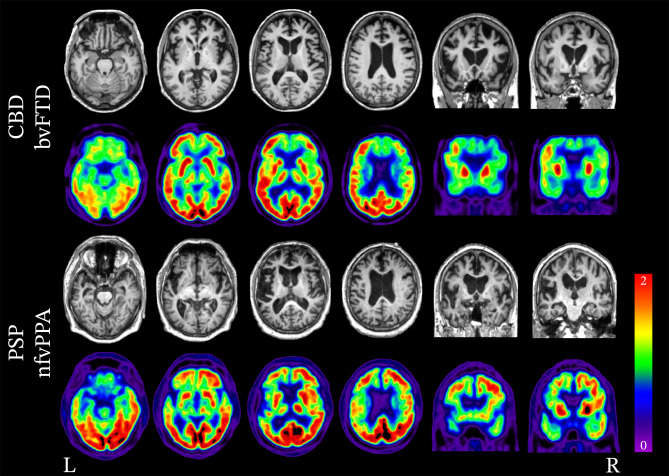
Neuroimaging patterns associated with 4R tauopathies (FTLD-tau). Structural MRI and FDG-PET demonstrating the variability in patterns of atrophy and hypometabolism attributed to 4R tauopathies, CBD and PSP
